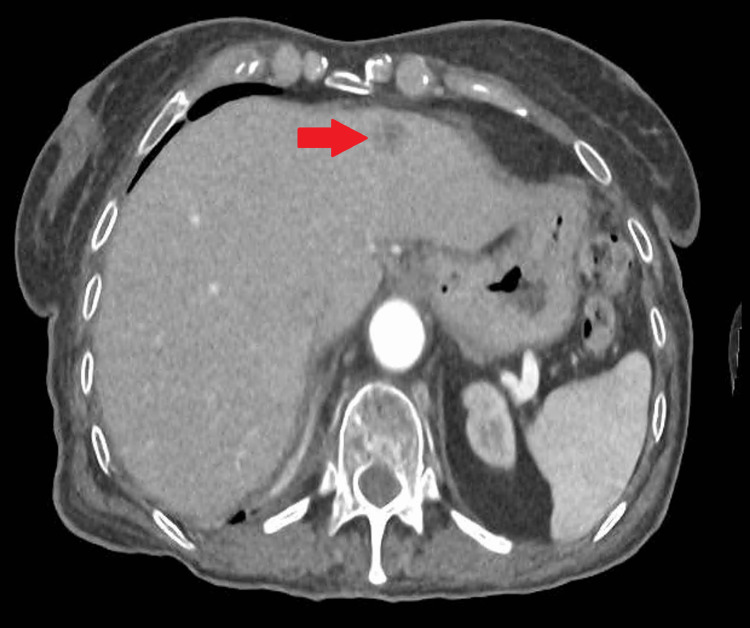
December 2023 CT scan showing disease progression with new hepatic lesions This image corresponds to the appearance of new metastatic liver lesions after receiving three cycles of pemetrexed. The red arrow corresponds to a new 13-mm metastatic lesion in segment III.
